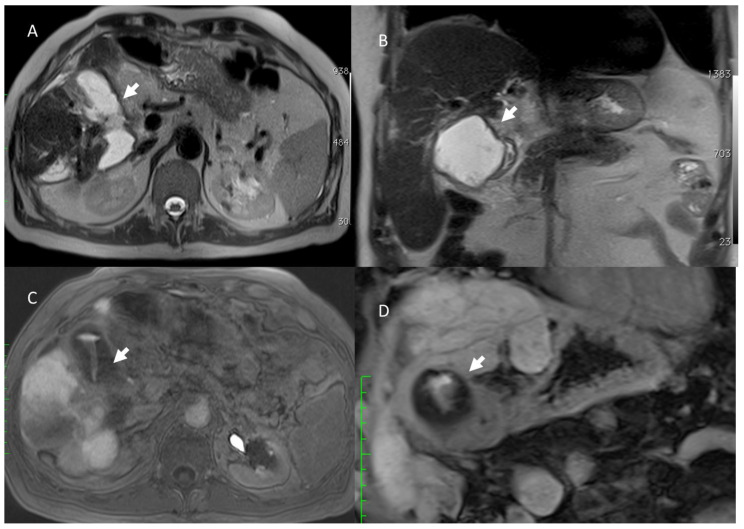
Man 74 years at 1-month follow-up after radio frequency of HCC on VI segment. MRI ((A,B) HASTE T2-weighted sequences in axial and coronal plane, in (C,D) EOB-phase of contrast study in axial and coronal plane). The arrow shows bile leak.
